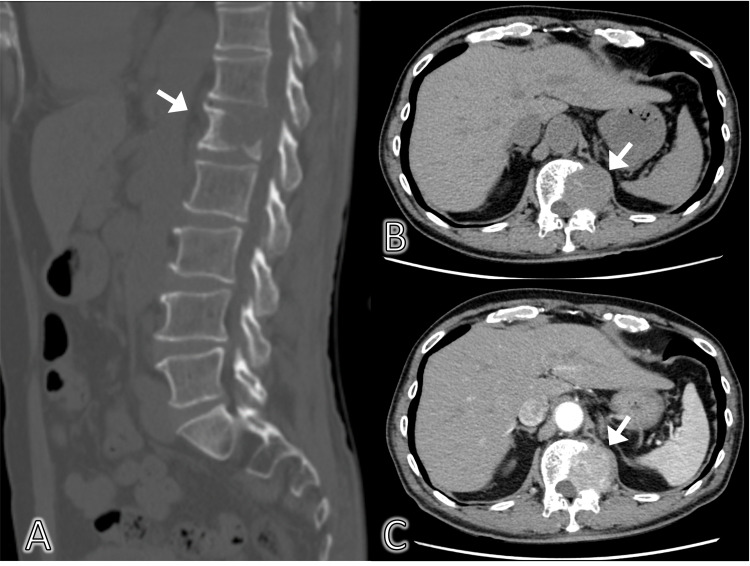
Abdominal CT (A. Sagittal plane, B. Coronal plain, C. Coronal plain with contrast). The osteolytic lesion in the first lumbar vertebra invading the left trabeculae on plain and enhanced CT.
